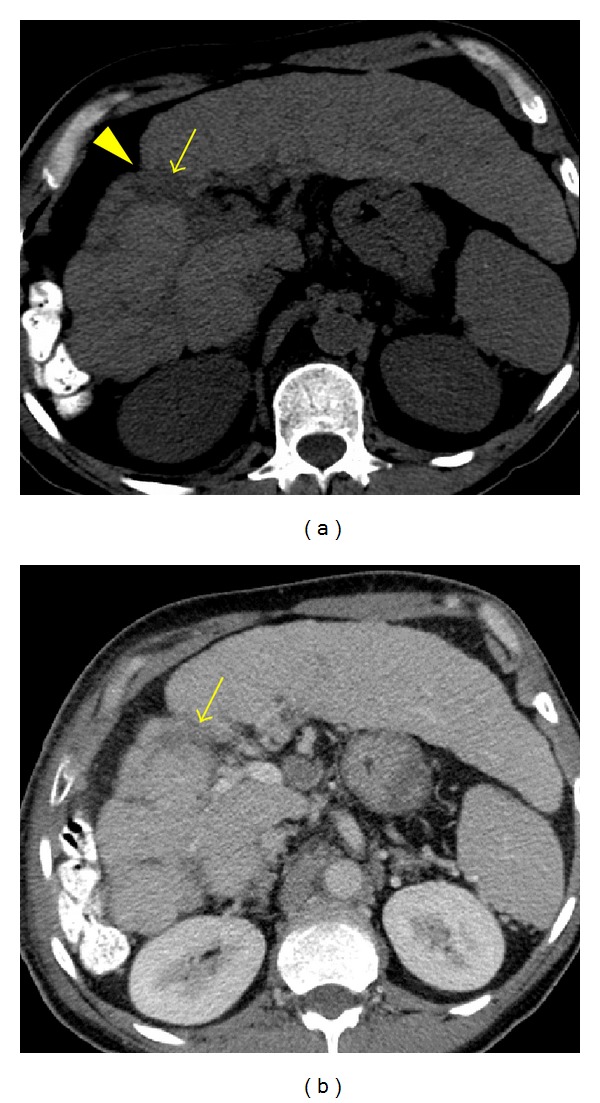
Confluent fibrosis in a 55-year-old male with alcoholic cirrhosis. (a) An unenhanced axial CT image shows a v-shaped area of subtle hypoattenuation (arrow) in hepatic segment 5. Note the retraction of the hepatic contour (arrowhead). (b) A portal venous phase axial image obtained at the same level as image (a) reveals an area of decreased portal venous flow (arrow).
